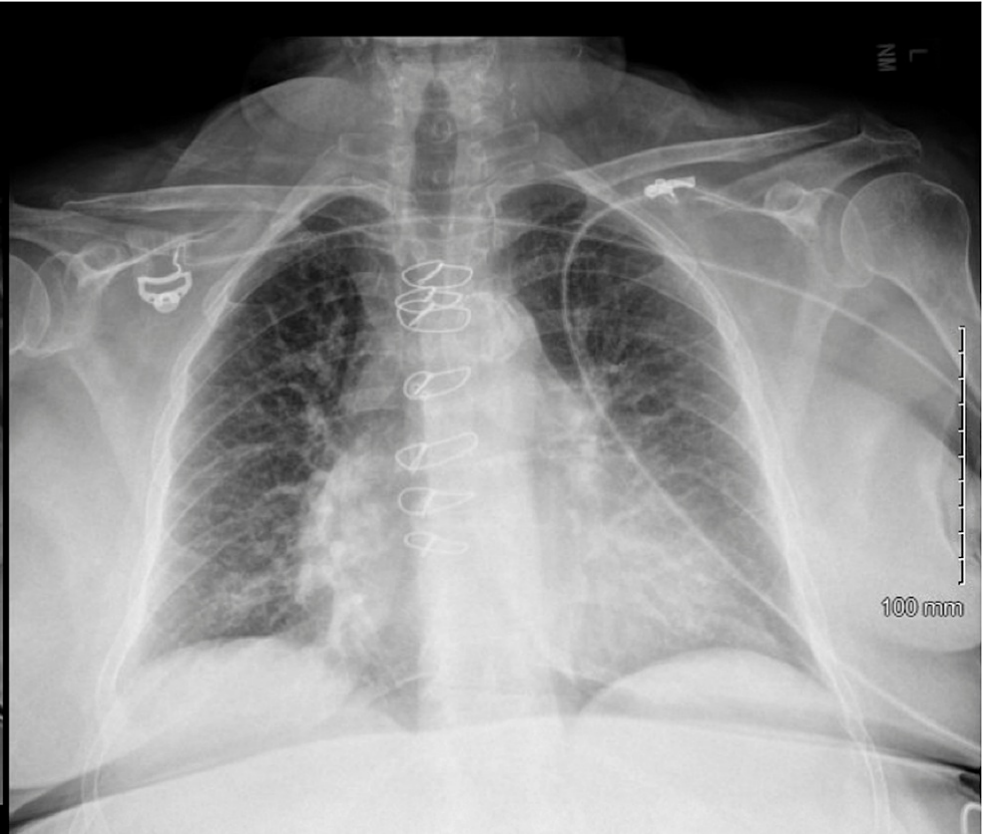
Chest X-ray before cardiac catheterization
